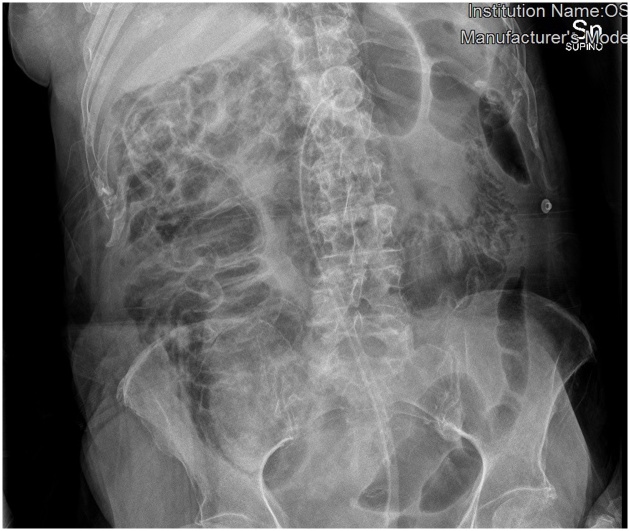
X-ray of abdomen shows important bowel distension.
